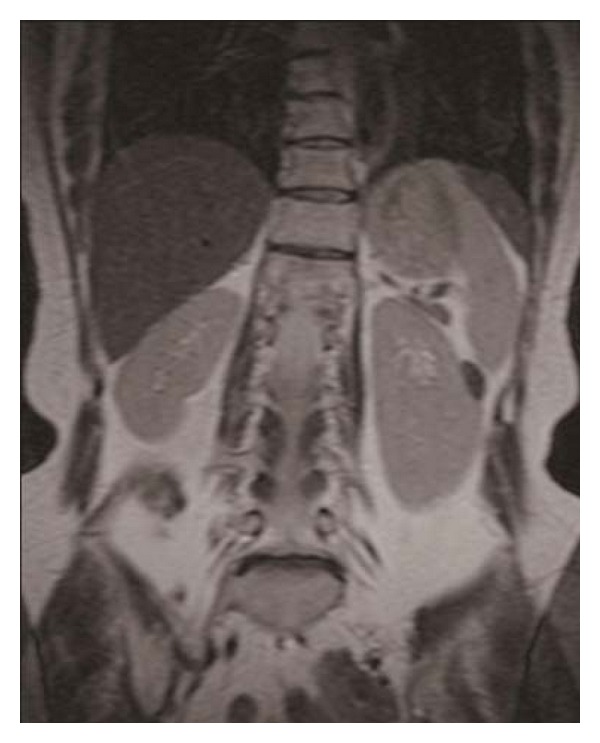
Coronal T1-weighted magnetic resonance imaging showing a tumor with homogenous low-signal intensity and well-defined margins.
